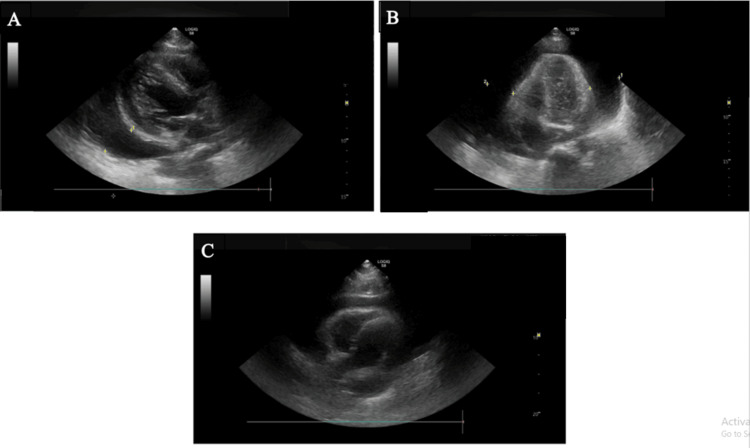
Representative transthoracic echocardiograms showing pericardial effusion in patients with non-cardiac effusion. (A, B) Moderate-to-large effusion with measured echo-free spaces; (C) large effusion surrounding the heart.
